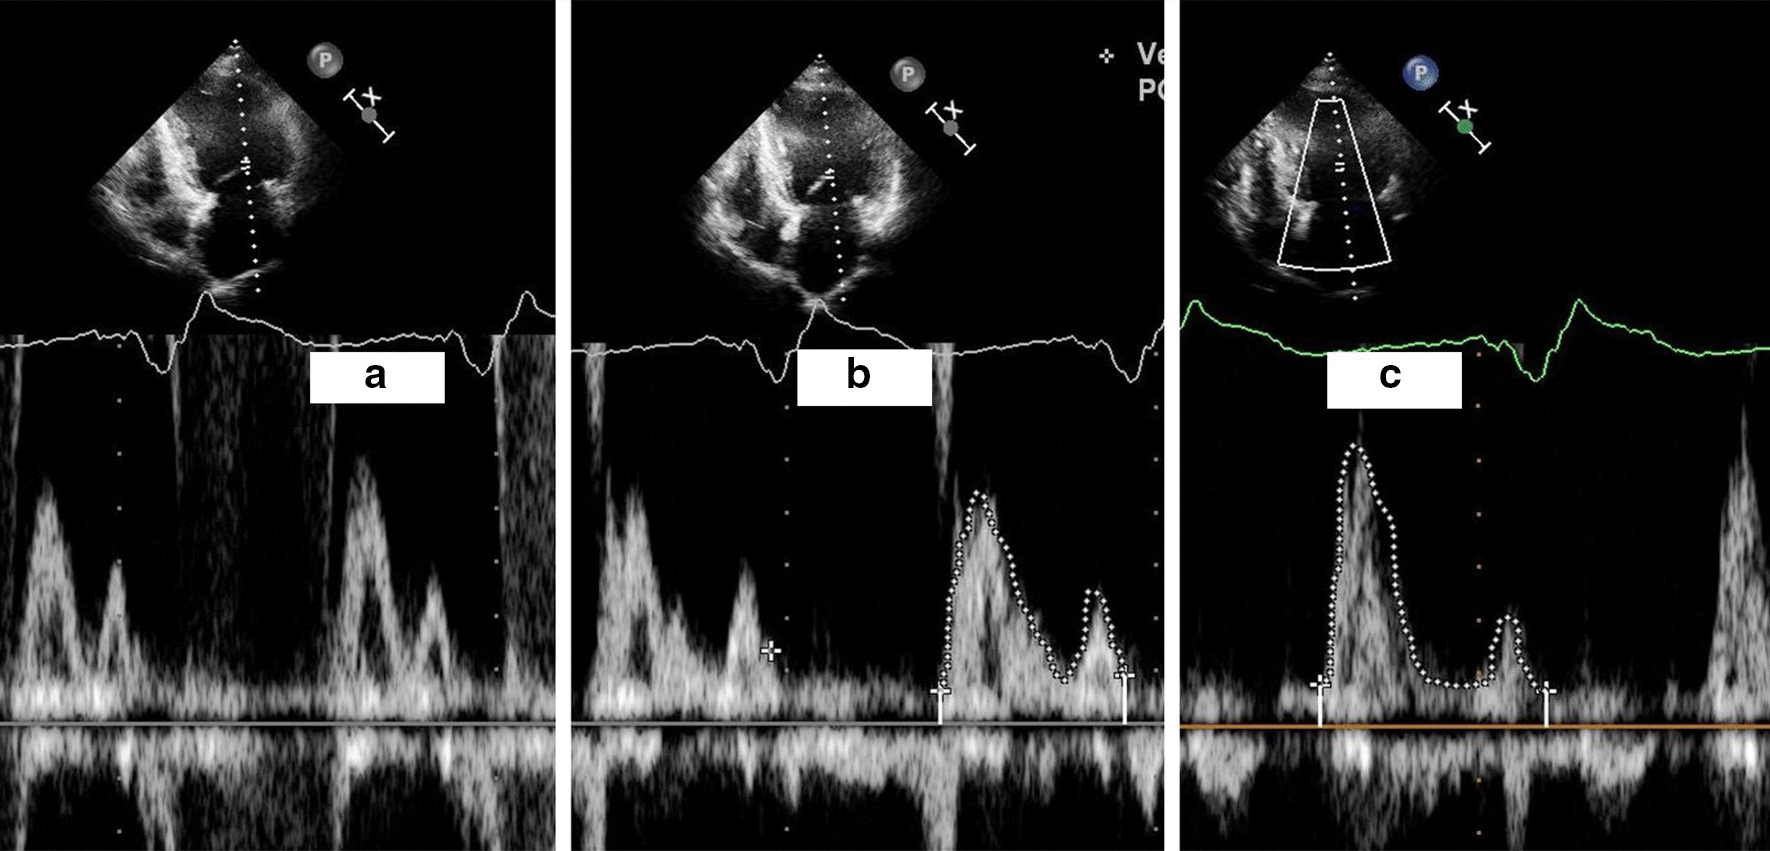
Mitral Doppler recordings. a EA fusion during ventricular pacing before new atrial lead implantation. b
